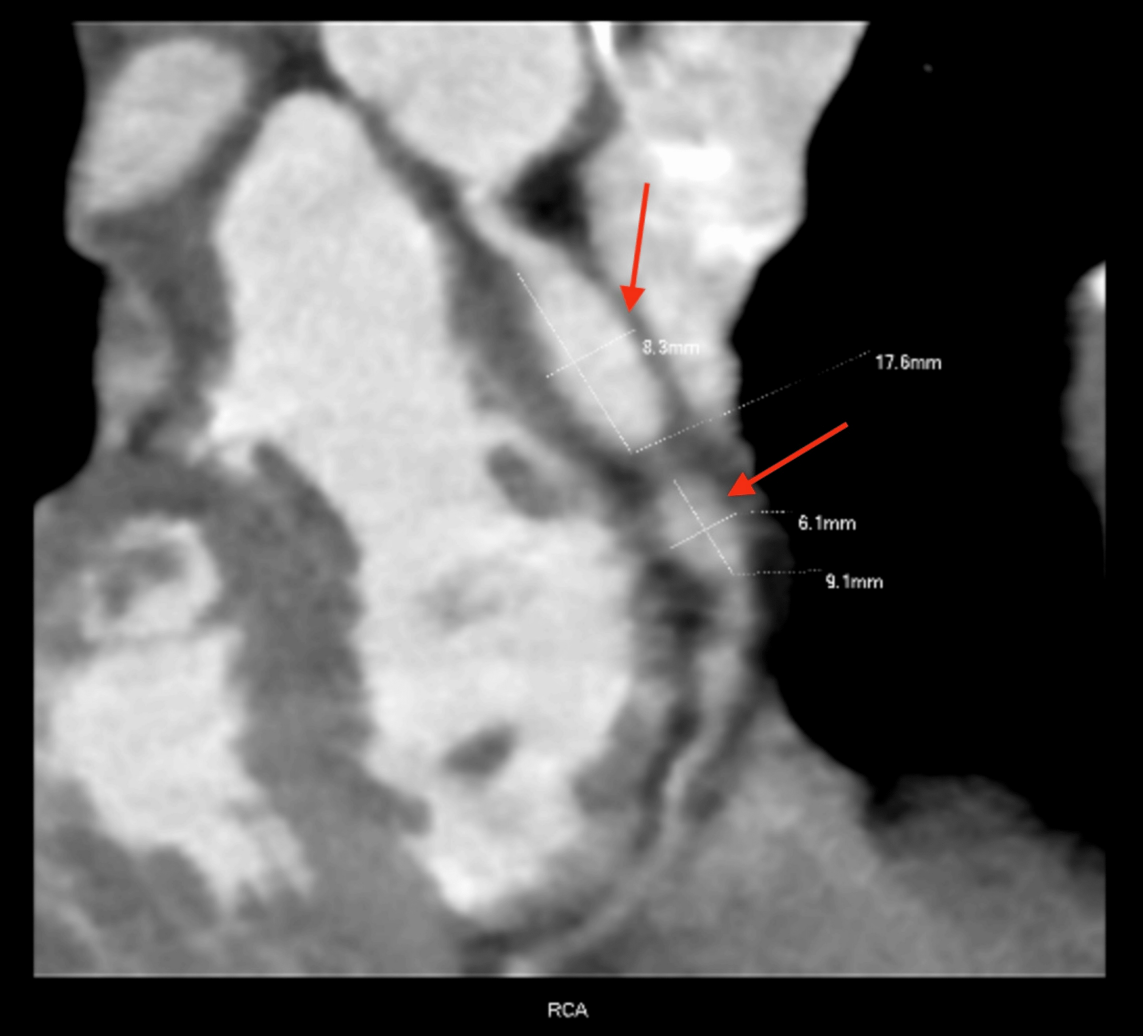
Coronary computed tomography angiography image of the patient The image is demonstrating multiple coronary artery aneurysms (red arrows).
